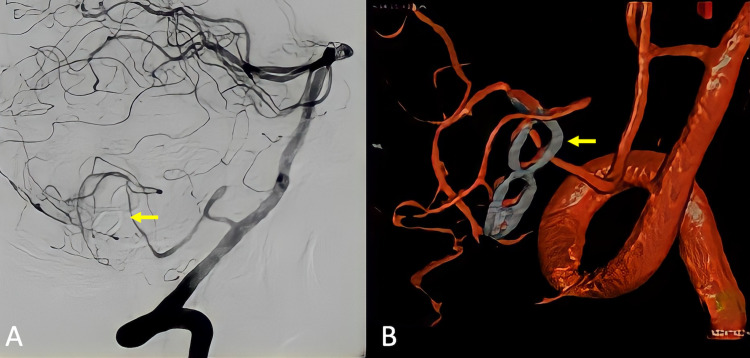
Postoperative imaging studies (A) Digital subtraction angiography and (B) CT angiography with 3D reconstruction showing complete aneurysm exclusion from the vertebrobasilar circulation and distal vessel's patency is confirmed. The 7-mm fenestrated clip can be observed occluding the aneurysm's neck (yellow arrows).
